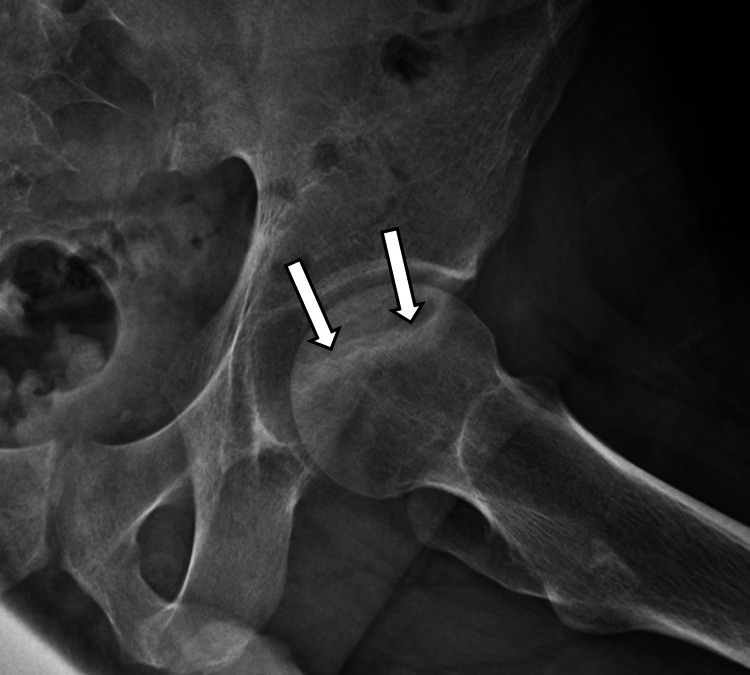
AP plain radiograph frog-leg view of the left hip Arrows pointing toward a sclerotic band on the left femoral head. AP: Anteroposterior
